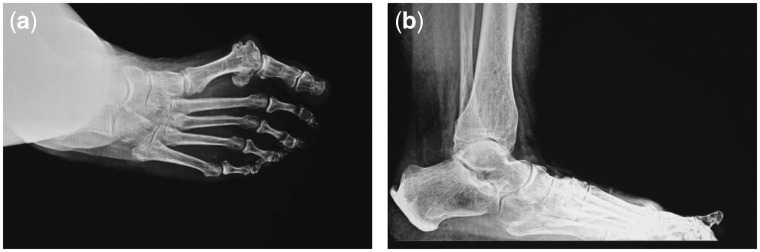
Transverse and sagittal section preoperative X-ray examination. Subluxated and collapsed medial arch was found out at the navicular-cuneiform, metatarsal-cuneiform joint with subluxed first MTPJ
